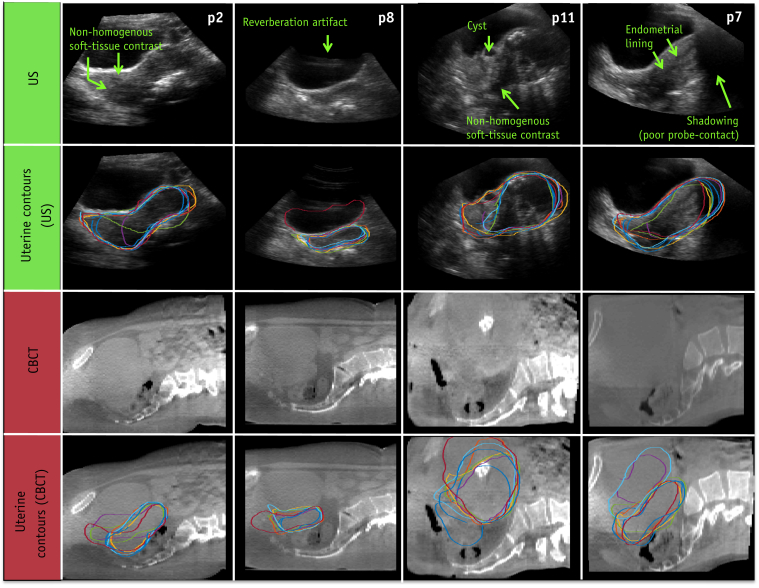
Examples of ultrasound (US) imaging features (row 1) that caused contouring disagreement (row 2) in patients 2, 8, 11, and 7 (p2-p11). Such features include nonhomogenous soft-tissue contrast in the uterus, internal structures such as cysts and the endometrial lining, and US artifacts such as shadowing and reverberations. Corresponding cone beam computed tomography (CBCT) and CBCT-based contours shown for reference in rows 3 and 4. The US and CBCT images are displayed on different scales (pixel sizes of [0.58, 0.58] mm and [2.5, 1] mm, respectively).
